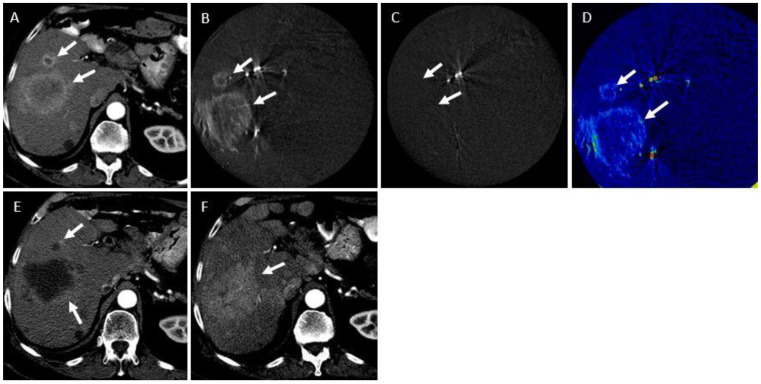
A 76-year-old male with hepatocellular carcinoma (HCC) presents for hepatic artery embolization. (A) Pre-procedural triphasic CT demonstrates two segment 8 lesions (7.6 cm and 1.7 cm in maximum axial diameter; arrows) with characteristic arterial-phase hyperenhancement, consistent with HCC. (B) Pre-embolization intraprocedural subtracted CBCT demonstrating the two hyperenhancing lesions corresponding to those on pre-procedural CT (arrows). (C) Post-embolization intraprocedural subtracted CBCT demonstrating complete absence of tumor enhancement in either lesion (arrows). (D) ∆ATE map demonstrates a ∆ATE of 20% for the large lesion and 23% for the smaller lesion. (E) One-month follow-up triphasic CT demonstrates residual areas of hyperenhancement on the arterial phase (arrow), which also wash out on the portal venous phase, consistent with residual HCC. Per mRECIST, treatment response was categorized as partial response (PR). (F) Three-month follow-up triphasic CT demonstrates local progression of disease (arrow).
